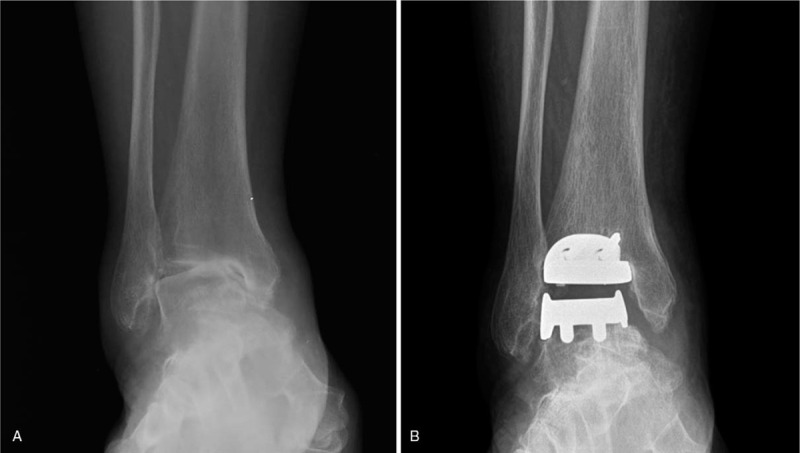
Weight-bearing anteroposterior radiographs of a 63-year-old woman. Preoperative radiograph indicating 21° of ankle incongruent varus deformity (A). Postoperative radiograph (B).
